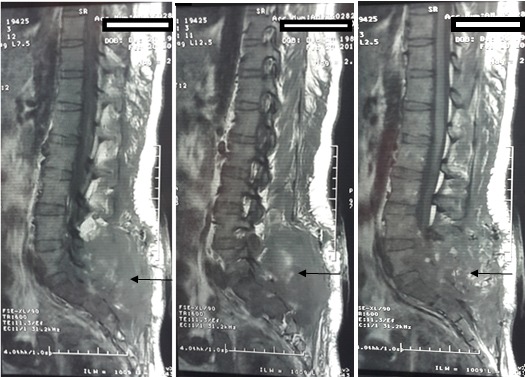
Postperative MRI shows lesion with low signal in T1WI and heterogeneous high signal in T2WI
